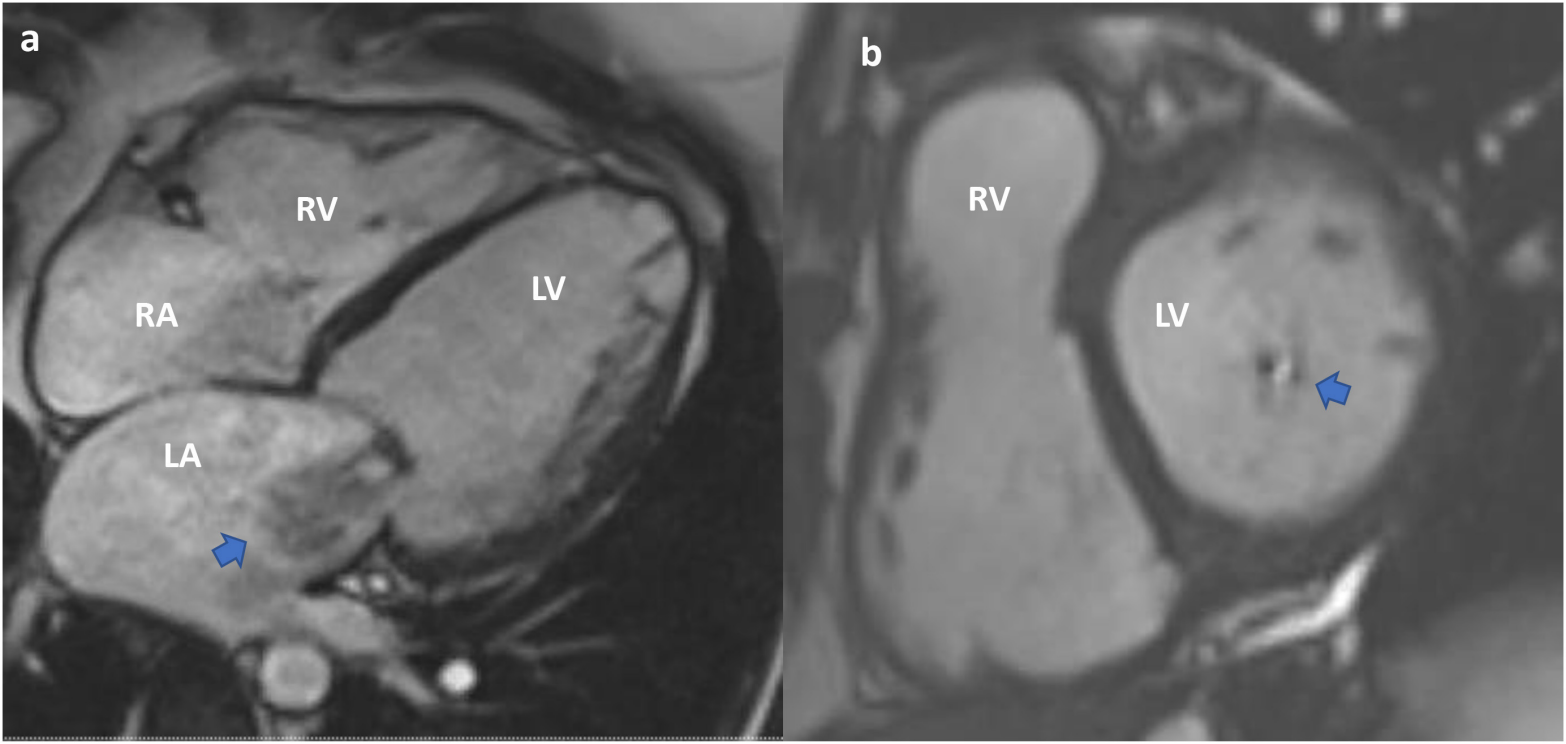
Cardiac MRI. Functional mitral regurgitation planning for mitral valve clip. (a) SSFP Cine 4-chamber showing severely dilated left ventricular cavity with “tented” mitral valve leaflets and evidence of significant mitral regurgitation (arrow) into a dilated left atrium. (b) SSFP Cine short axis of the mitral valve closure showing the jet of mitral regurgitation mostly confined toward the posterior annulus (arrow).
